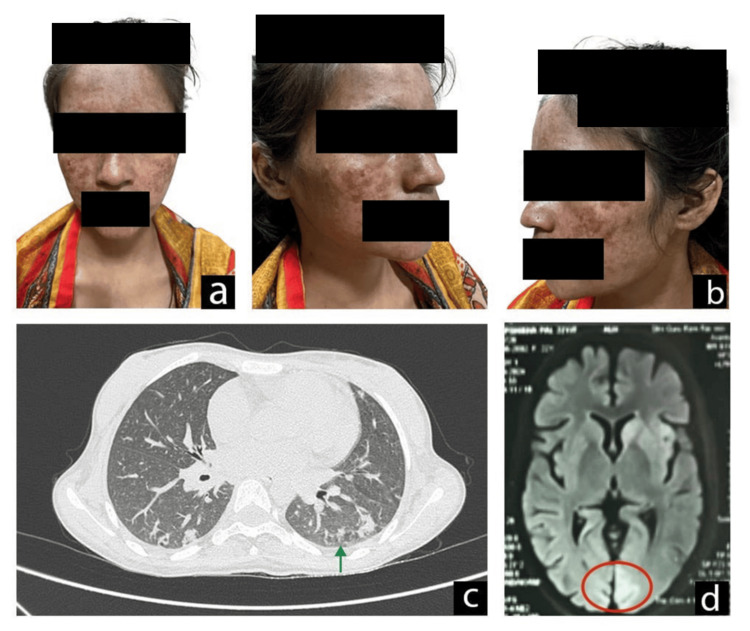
During the episode of systemic exacerbation (a and b) Facial profile of the patient, (c) high-resolution computed tomography (HRCT) of the thorax showing a nonspecific interstitial pneumonitis (NSIP) pattern (green arrow). (d) MRI brain image showing near symmetrical areas of faint signal alteration in bilateral occipital regions suggestive of Posterior Reversible Encephalopathy Syndrome (PRES) (red circle).
